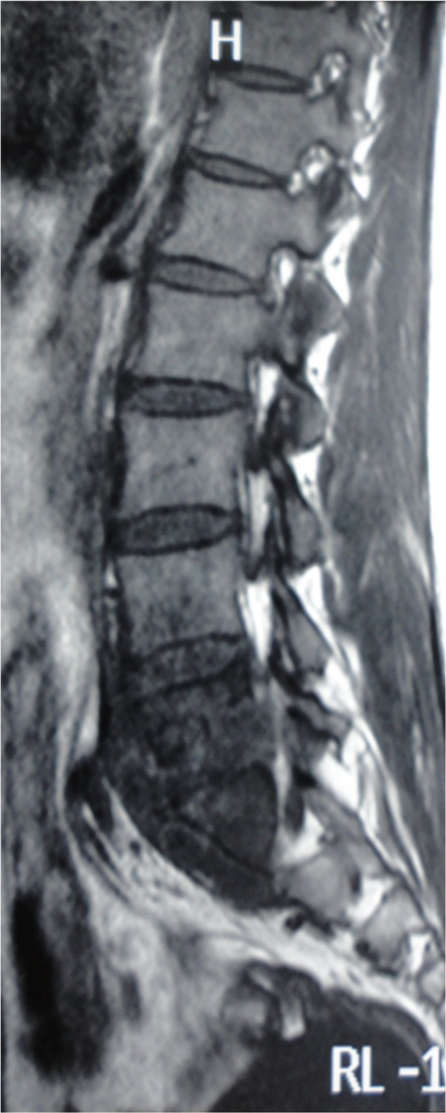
Preoperative magnetic resonance image of a 23 year old female patient with lumbosacral tuberculosis
